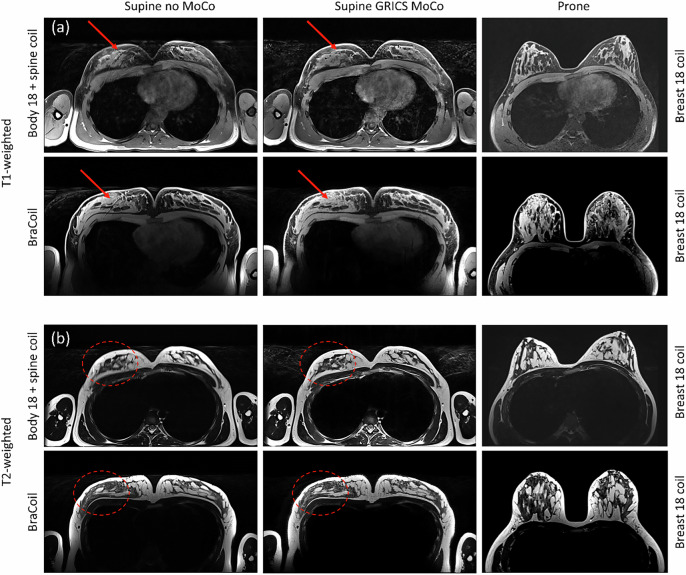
a T1- and (b) T2-weighted images with BraCoil *versus* Body18 coil with (central column) and without (left column) motion correction (MoCo). The right column shows the corresponding prone images for comparison. Prone images have been zero-padded for aesthetic purposes. The coils used for the supine position are indicated on the left, and the coil used for the prone position is noted on the right. The red arrows point to the ghosting artifacts corrected by MoCO, and the red dashed ellipses indicate the regions where the sharpness was improved after the motion correction
